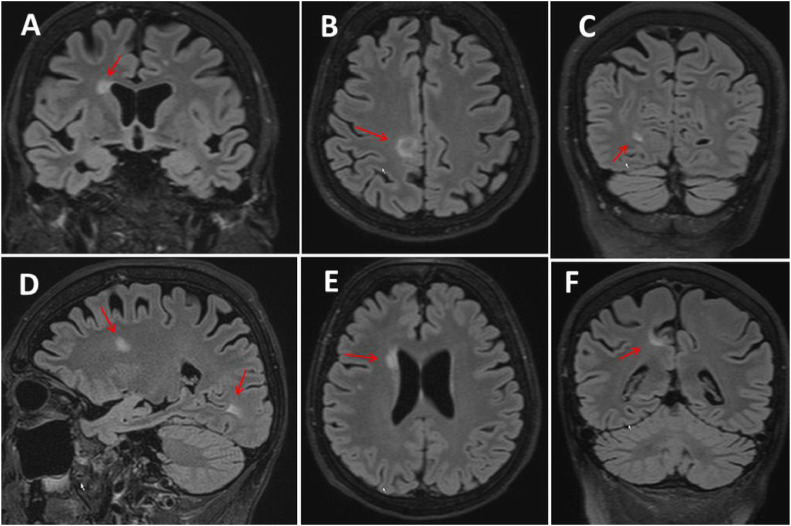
Cerebral MRI from January 2024 showing multiple T2 hyperintense lesions in the right hemisphere, especially in the right frontal lobe perpendicularly (panels A, D, E), in the parietal lobe (panels B, F), and the right occipital lobe (panels C, D).
